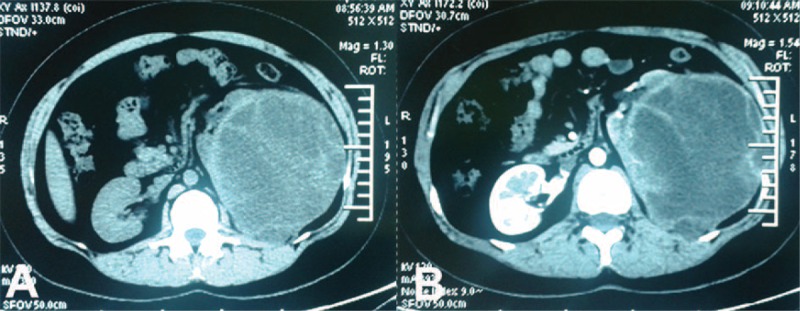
(A) Computer tomography (CT) scan revealed a 14.7 × 12.7 cm well-defined, unevenly mass lesion with both solid and cystic components. (B) The tumor was not enhanced uniformly.
